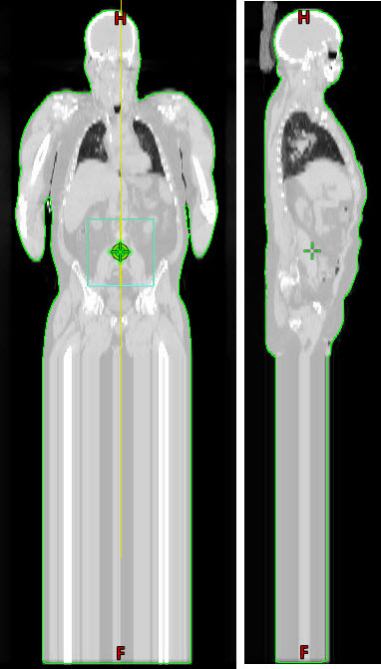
Example of a patient CT scan with the mid‐thigh position extended to the height of the patient.
